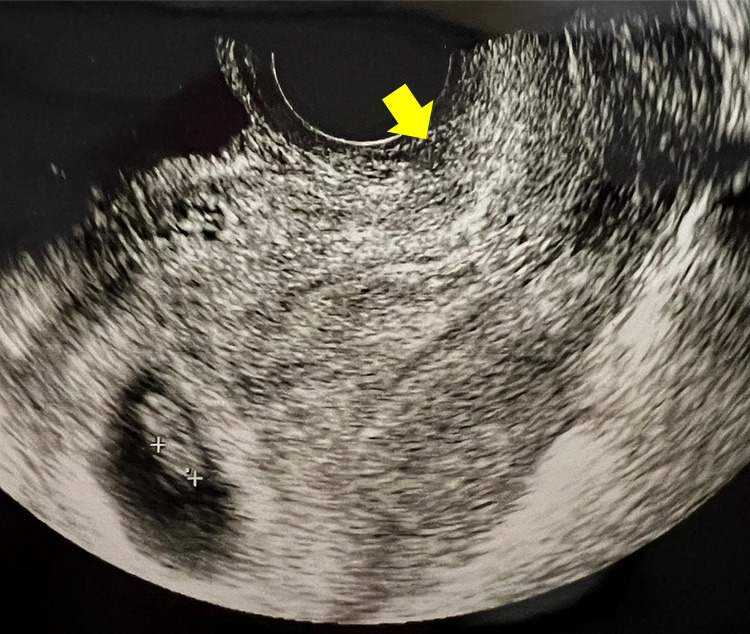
Ultrasound: RMT area during spontaneous pregnancy (arrow)
